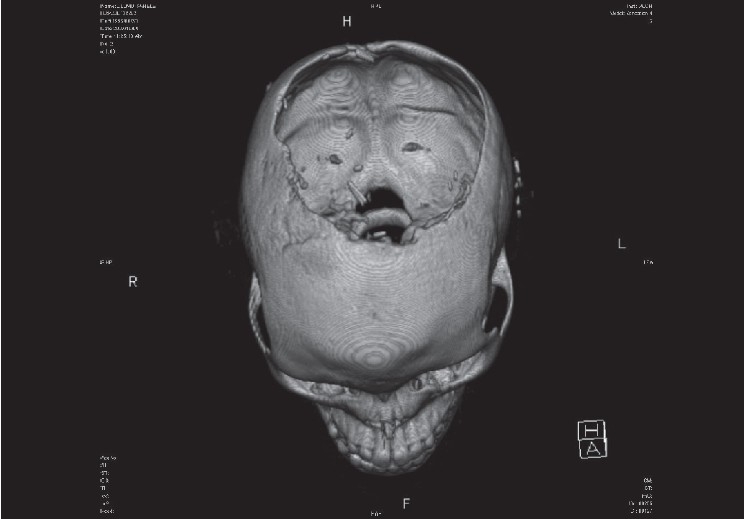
CT scan showing a large cranial bone defect due to erosion by tumour
